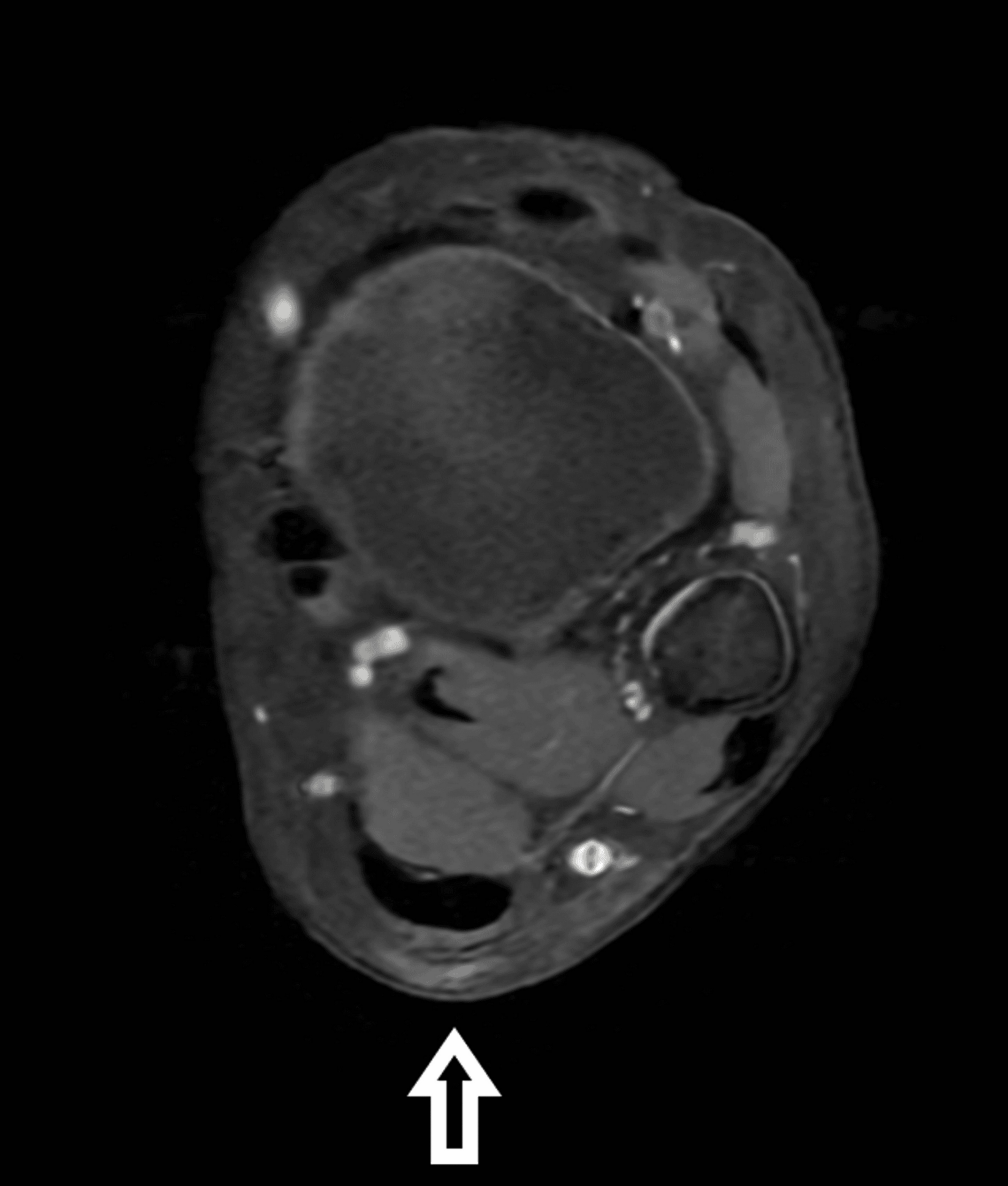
MRI scan on the transverse plane showing a soft tissue subcutaneous mass overlying the lower third of the Achilles tendon, with areas of lower signal intensity on T1.
